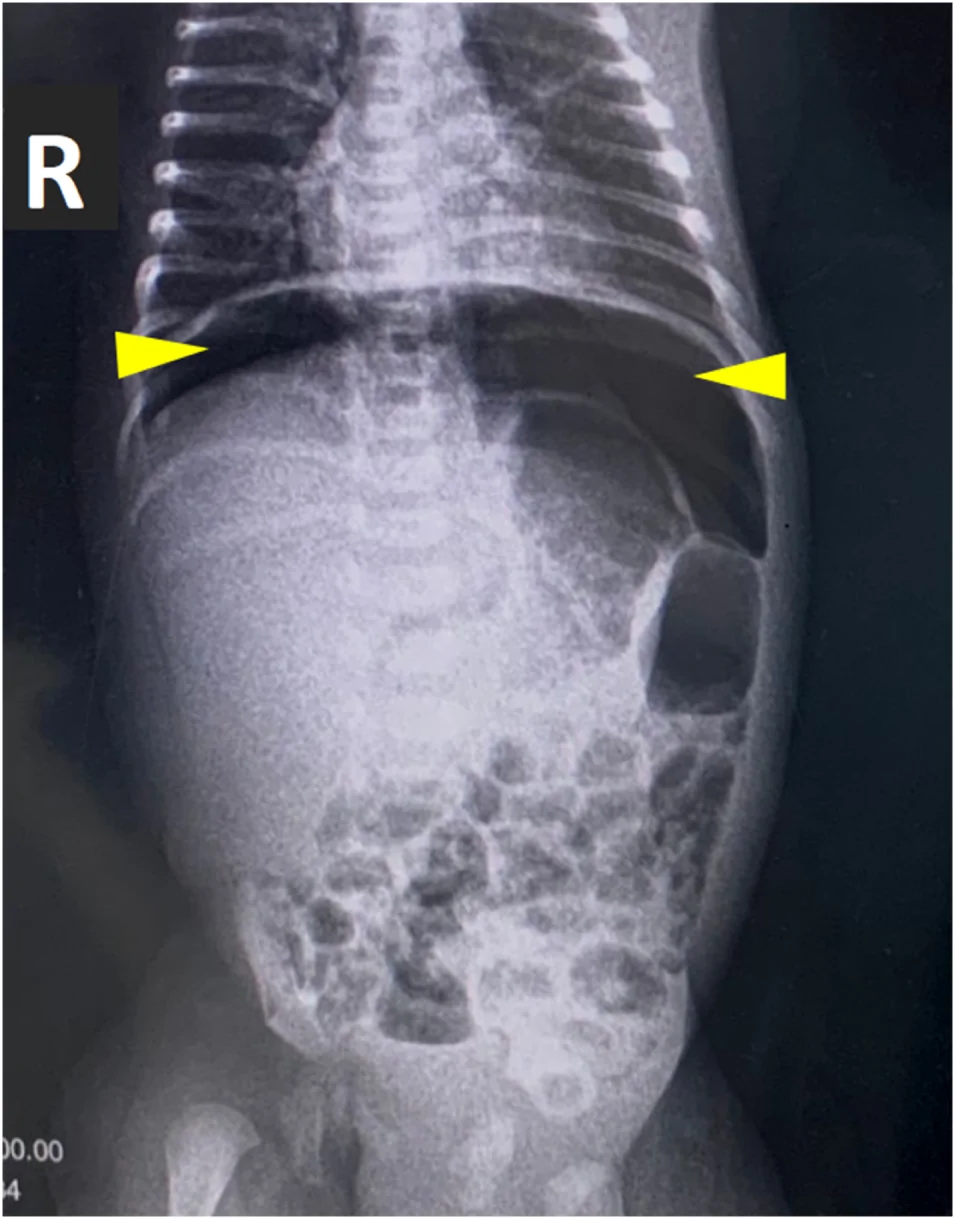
Plain abdominal x-ray. Note significant pneumoperitoneum under both sides of the diaphragmatic domes (yellow arrows) and no pulmonary disease.
